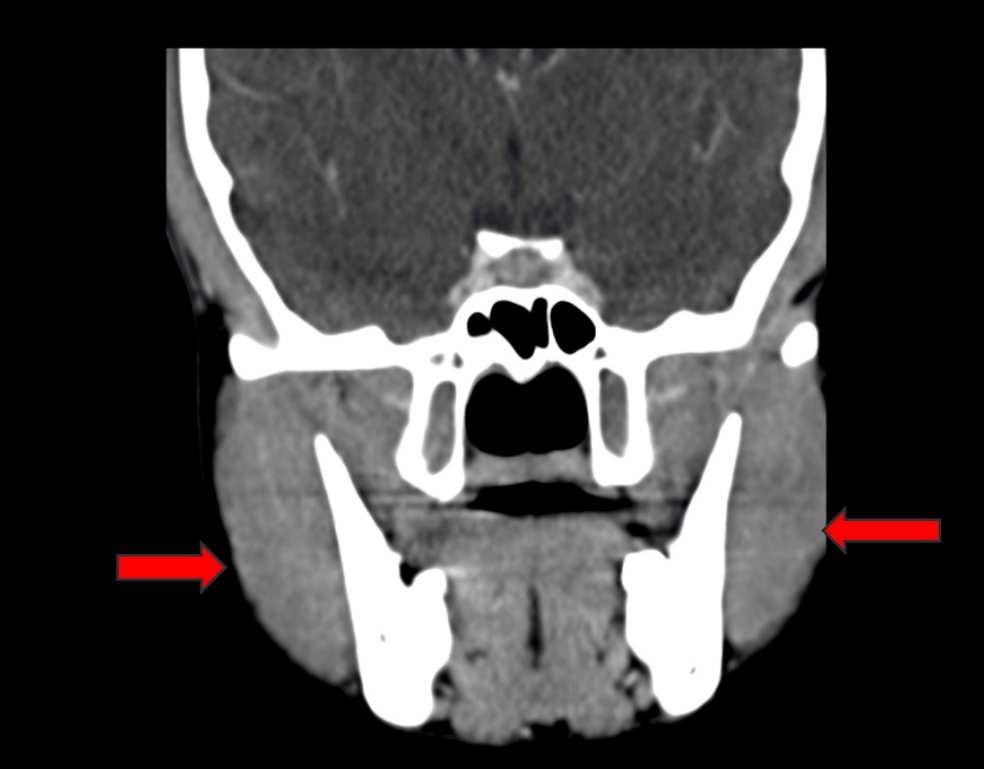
CT scan coronal view showing bilateral masseter muscle hypertrophy (red arrows)
